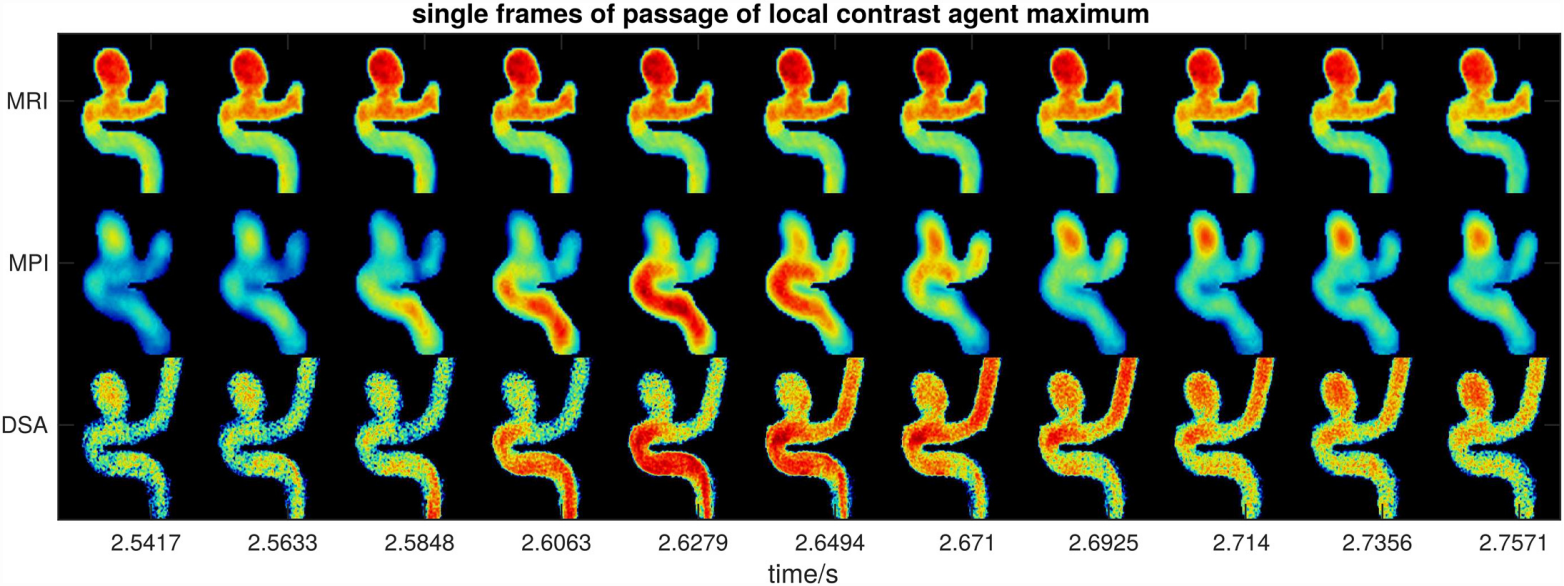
Multiple frames of dynamic MRI, MPI, and DSA measurements during the passage of a local maximum of contrast agent concentration (see time points 2.5 s-2.8 s in Fig 2). The passage of the contrast agent bolus through the main vessel of the model can be estimated in MPI and DSA, but not in MRI. A movie showing all frames of the dynamic scans is available as S3 Video.
